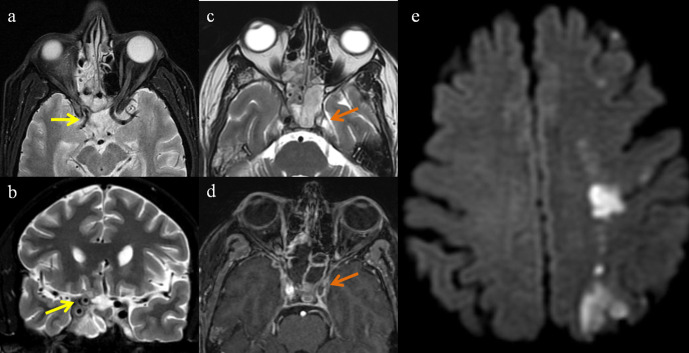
Axial (a) and coronal (b) T2FS images of mucormycosis showing vessel wall irregularity and thickening (yellow arrow) indicating vasculitis. T2 Axial (c), postcontrast axial T1FS (d) and DWI (e) images of another patient with mucormycosis showing angioinvasion. (c) T2W image showing ethmoid and sphenoid sinusitis with loss of flow void in left ICA (arrow).(d) Postcontrast T1W image shows no enhancement of left ICA (arrow). (e) DWI image shows superficial and deep watershed territory infarcts in left cerebral hemisphere.
